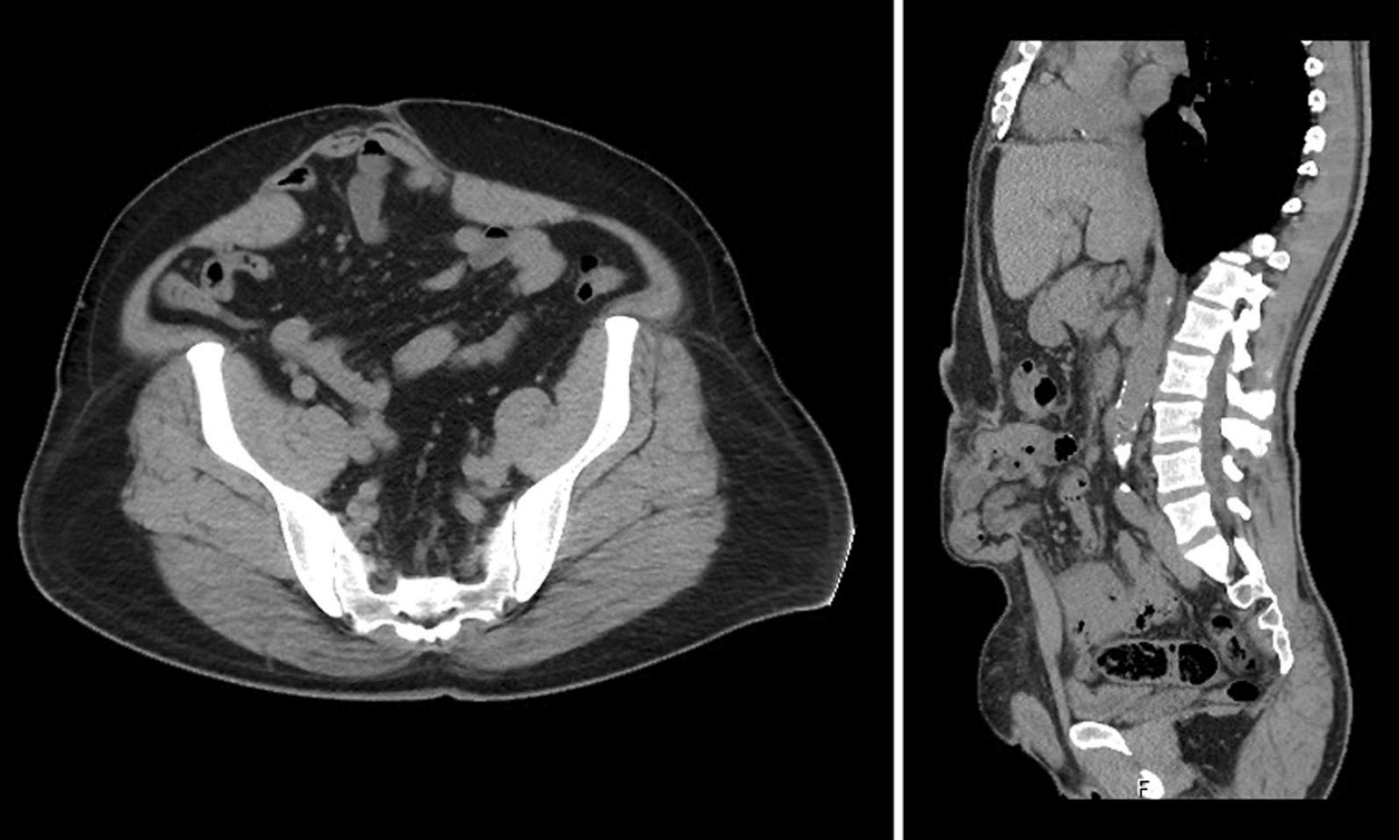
Abdominal CT shows an incisional hernia orifice in the infra-umbilical region (M3, M4)
